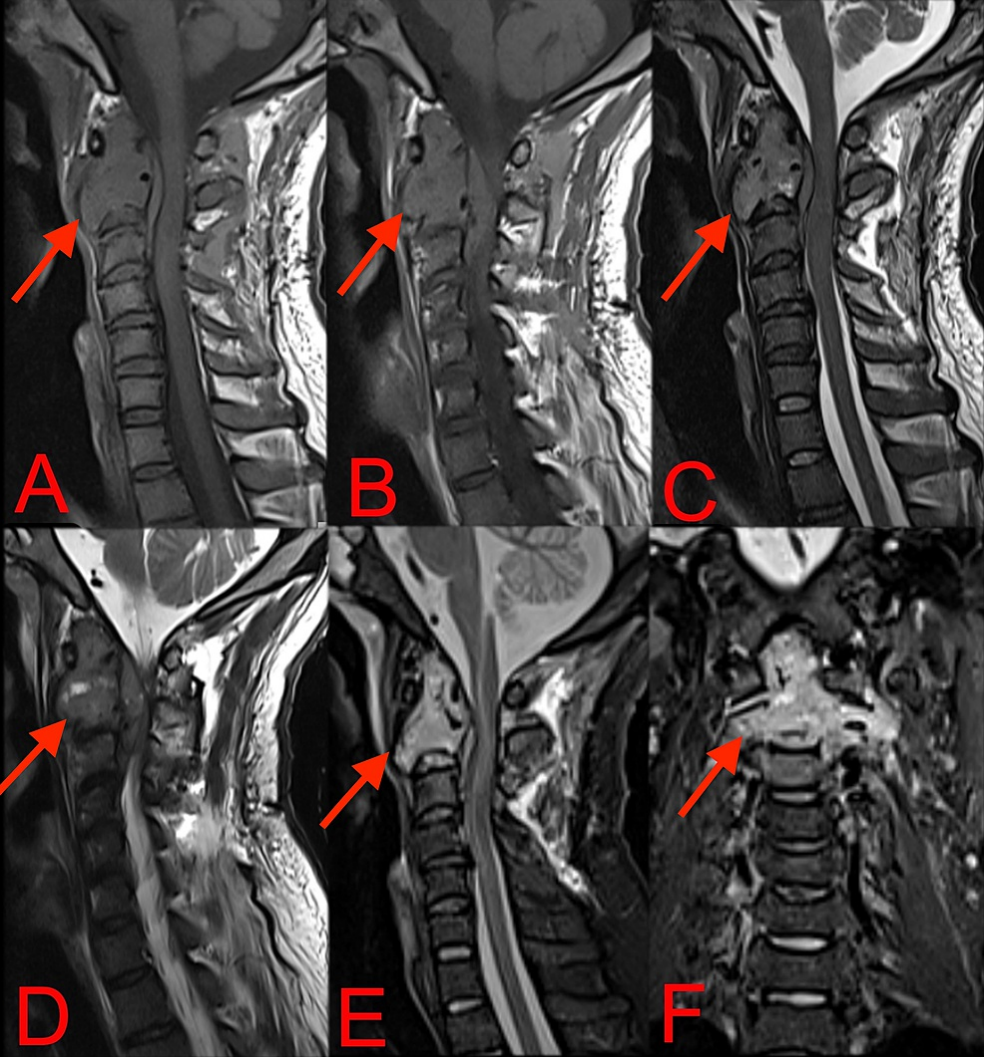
T1 (A, B) and T2 (C, D) weighted mid-sagittal and parasagittal MRI sections and sagittal and coronal STIR (E, F) images showed a lesion which is isointense to muscle on T1 and hyperintense on T2, causing compression of spinal cord with associated cord edema and near-total involvement of the C2 vertebra. MRI: Magnetic Resonance Imaging, STIR: short tau inversion recovery
